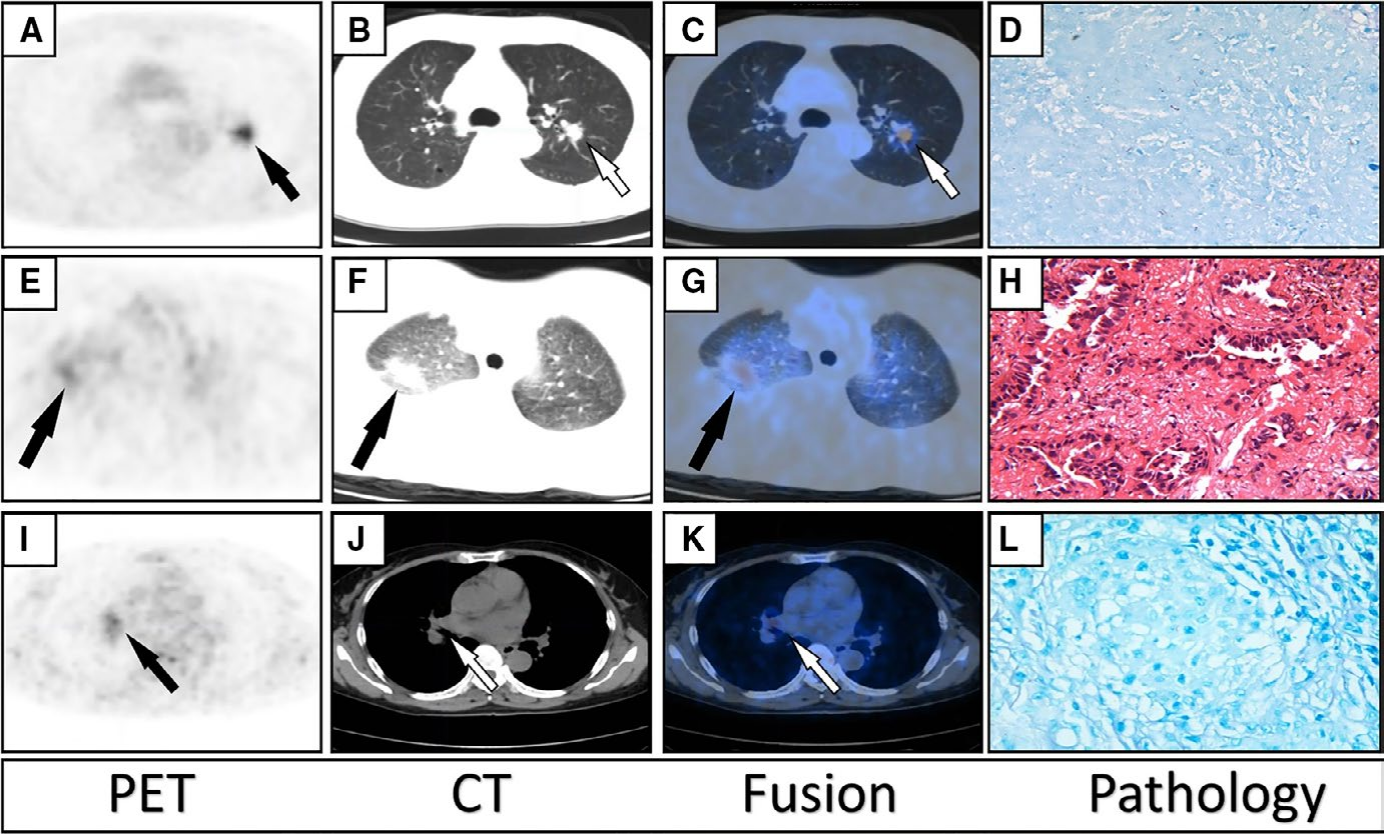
A–D, axial PET, CT, fused PET/CT images, and pathology of a 53 year‐old‐male patient diagnosed with PTB on ^18^F‐FDG PET/CT in the upper lobe of the left lung and confirmed by pathology analysis as PTB (true‐positive PTB); SUVmax: 6.1, SA: 1.5 cm, LA: 1.7 cm. E–H, axial PET, CT, fused PET/CT and pathology images of a 44‐year‐old female patient diagnosed as PTB on ^18^F‐FDG PET/CT in the middle lobe of the right lung with pathologic diagnosis of an adenocarcinoma, resulting in false‐positive PTB; SUVmax: 3.6, SA: 1.7cm, LA: 2.7 cm. I–L, axial PET, CT, fused PET/CT, and pathology images of a 51‐year‐old female patient diagnosed as lung cancer on ^18^F‐FDG PET/CT in the lower lobe of the right lung with a pathology diagnosis of PTB ( false‐negative PTB); SUVmax: 4, SA: 1.2 cm, LA: 1.4 cm. (Abbreviations: PET/CT, Positron Emission Tomography/Computed Tomography; FDG, Fluorine‐2‐Deoxy‐ D‐glucose; PTB, Pulmonary tuberculosis; SUVmax, Maximum Standardized Uptake value; SA, Short Axis, LA, Long axis)
